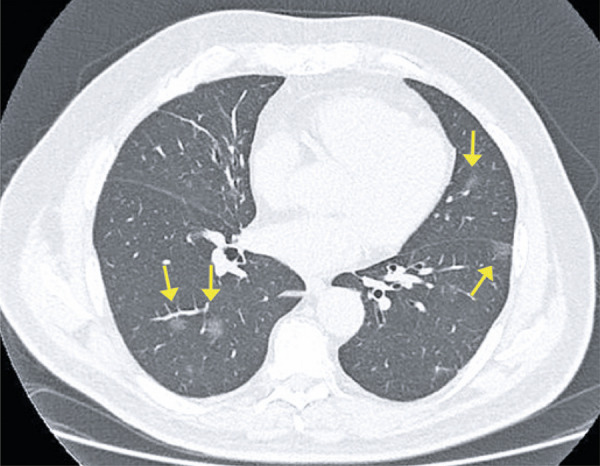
A 72-year-old patient with COVID-19, presenting cough, fever and dyspnea for four days. Computed tomography image show multifocal rounded ground-glass opacities, with typical aspect and distribution of COVID-19
